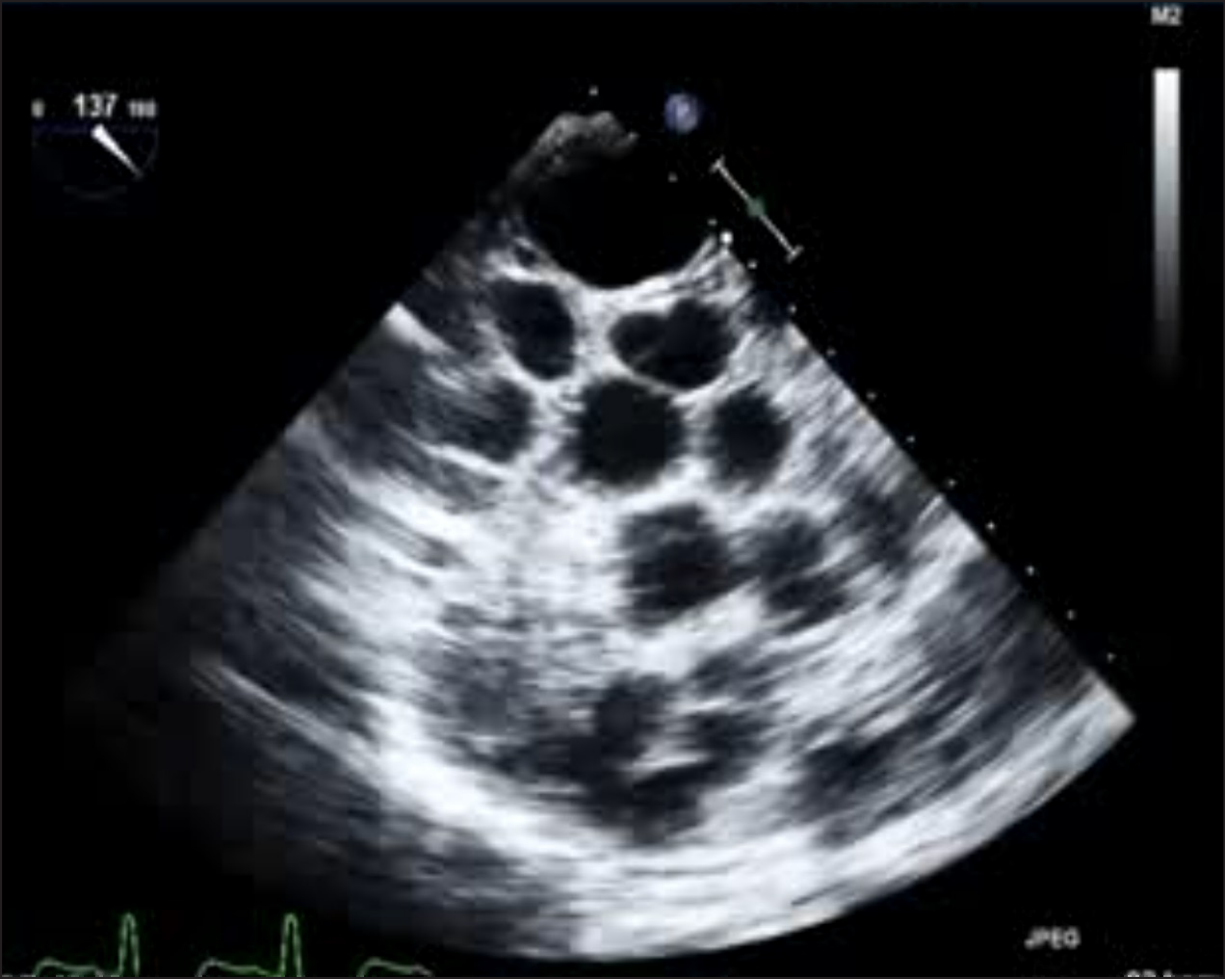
A transesophageal echocardiogram reveals the presence of numerous hydatid cysts entirely filling the left atrium and left ventricle cavity.
